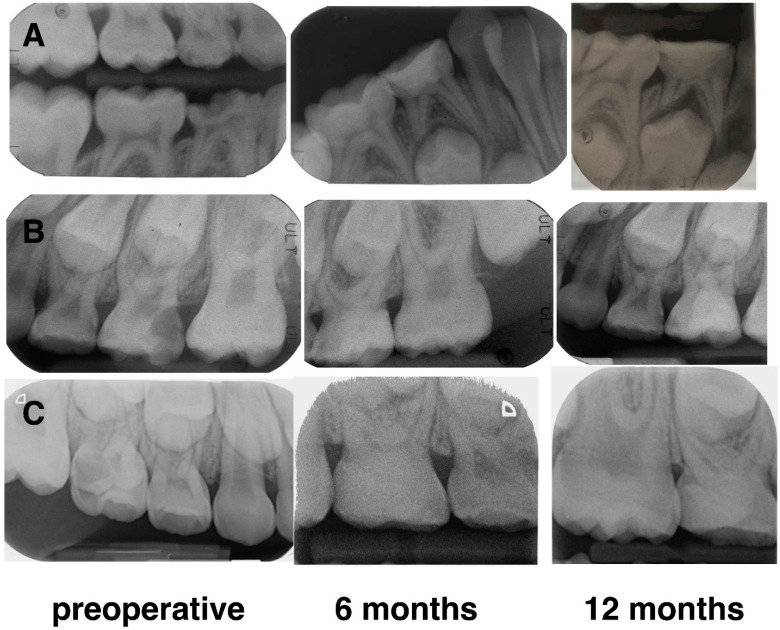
Radiographic images of successful pulpotomy of three primary molars at 6 and 12 months. Case (A): lower right first molar in stage S in a 6-year-old male; Case (B): upper left second molar in stage R in a 10-year-old female; Case (C): upper right second molar in stage S in a 6-year-old female.
